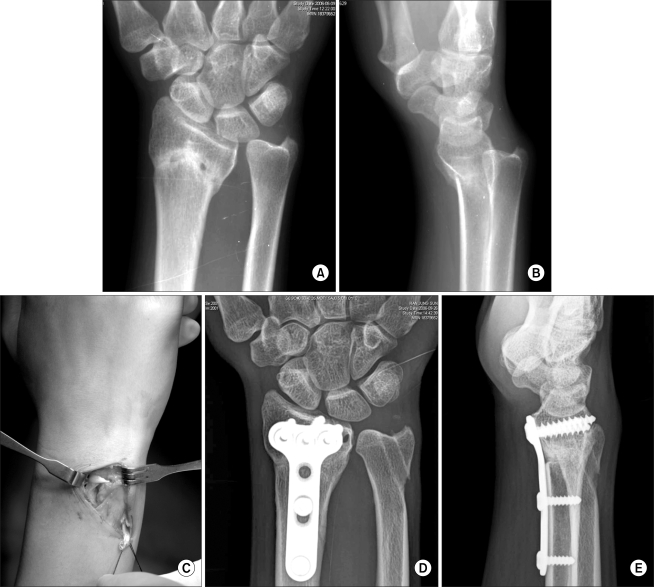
Preoperative radiographs of a 24 year-old woman shows distal radioulnar joint subluxation associated with radial malunion (A & B). This patient was treated with osteotomy and distal radioulnar ligament reconstruction using the palmaris longus tendon (C). Radiographs obtained two years after surgery show restored alignment. Note that the bone tunnels for ligament reconstruction in the distal radius and ulnar head are visible (D & E).
